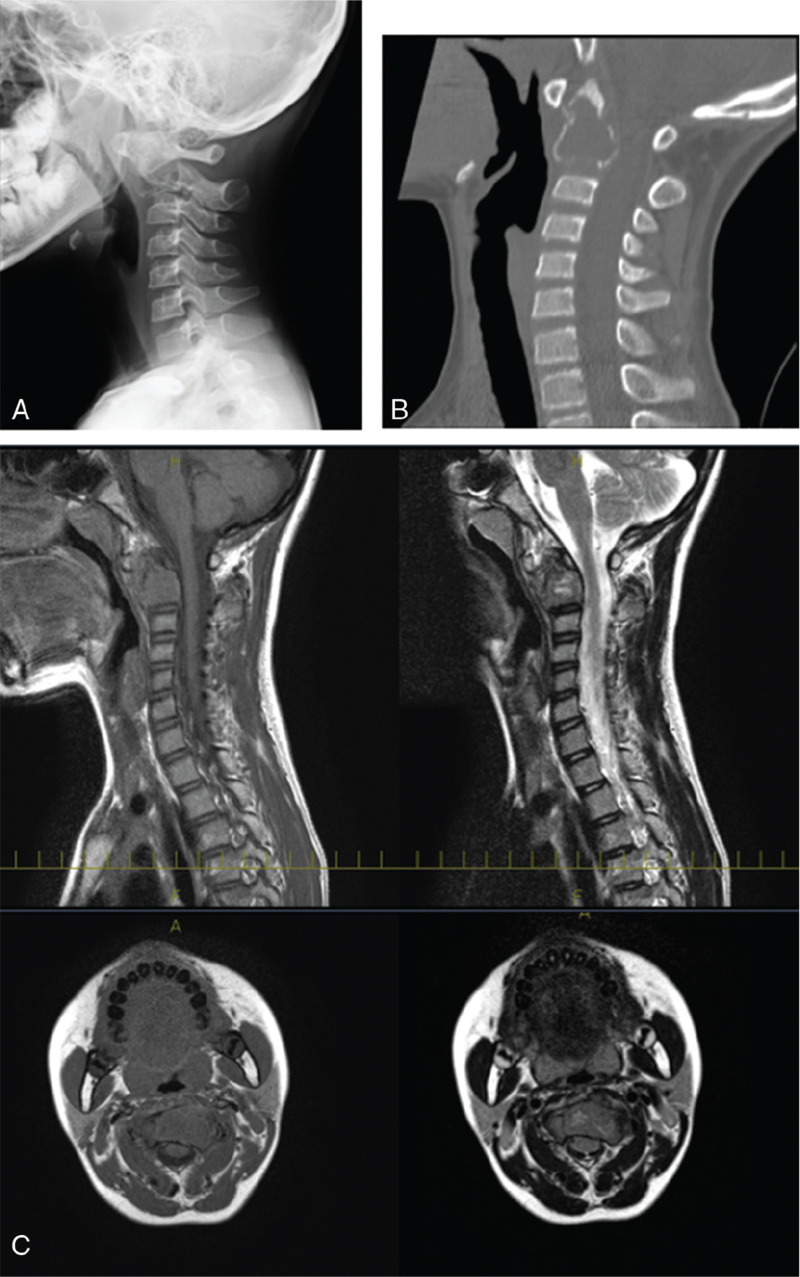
Radiological (A), computed tomography (B), and magnetic resonance (C) images during the first visit of the patient to our hospital (A). (A, B) Plain radiography and computed tomography show a radiolucent shadow and a mild osteolytic lesion of the C2 vertebral body. (C) Magnetic resonance imaging shows a mass of the C2 vertebral body, with low signal intensity on T1-weighted images and heterogeneous high signal intensity on T2-weighted images.
